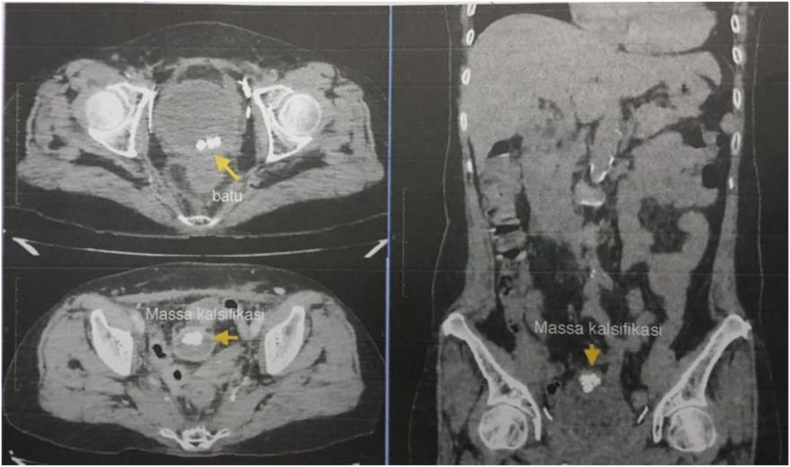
Non-contrast abdominal pelvic CT Scan.
